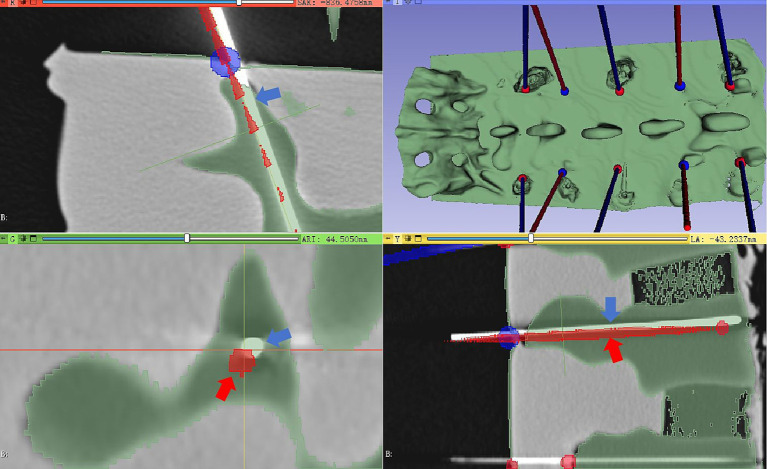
Registration of CT images: the blue arrow indicates the K-wire and the red arrow indicates the planned path.
